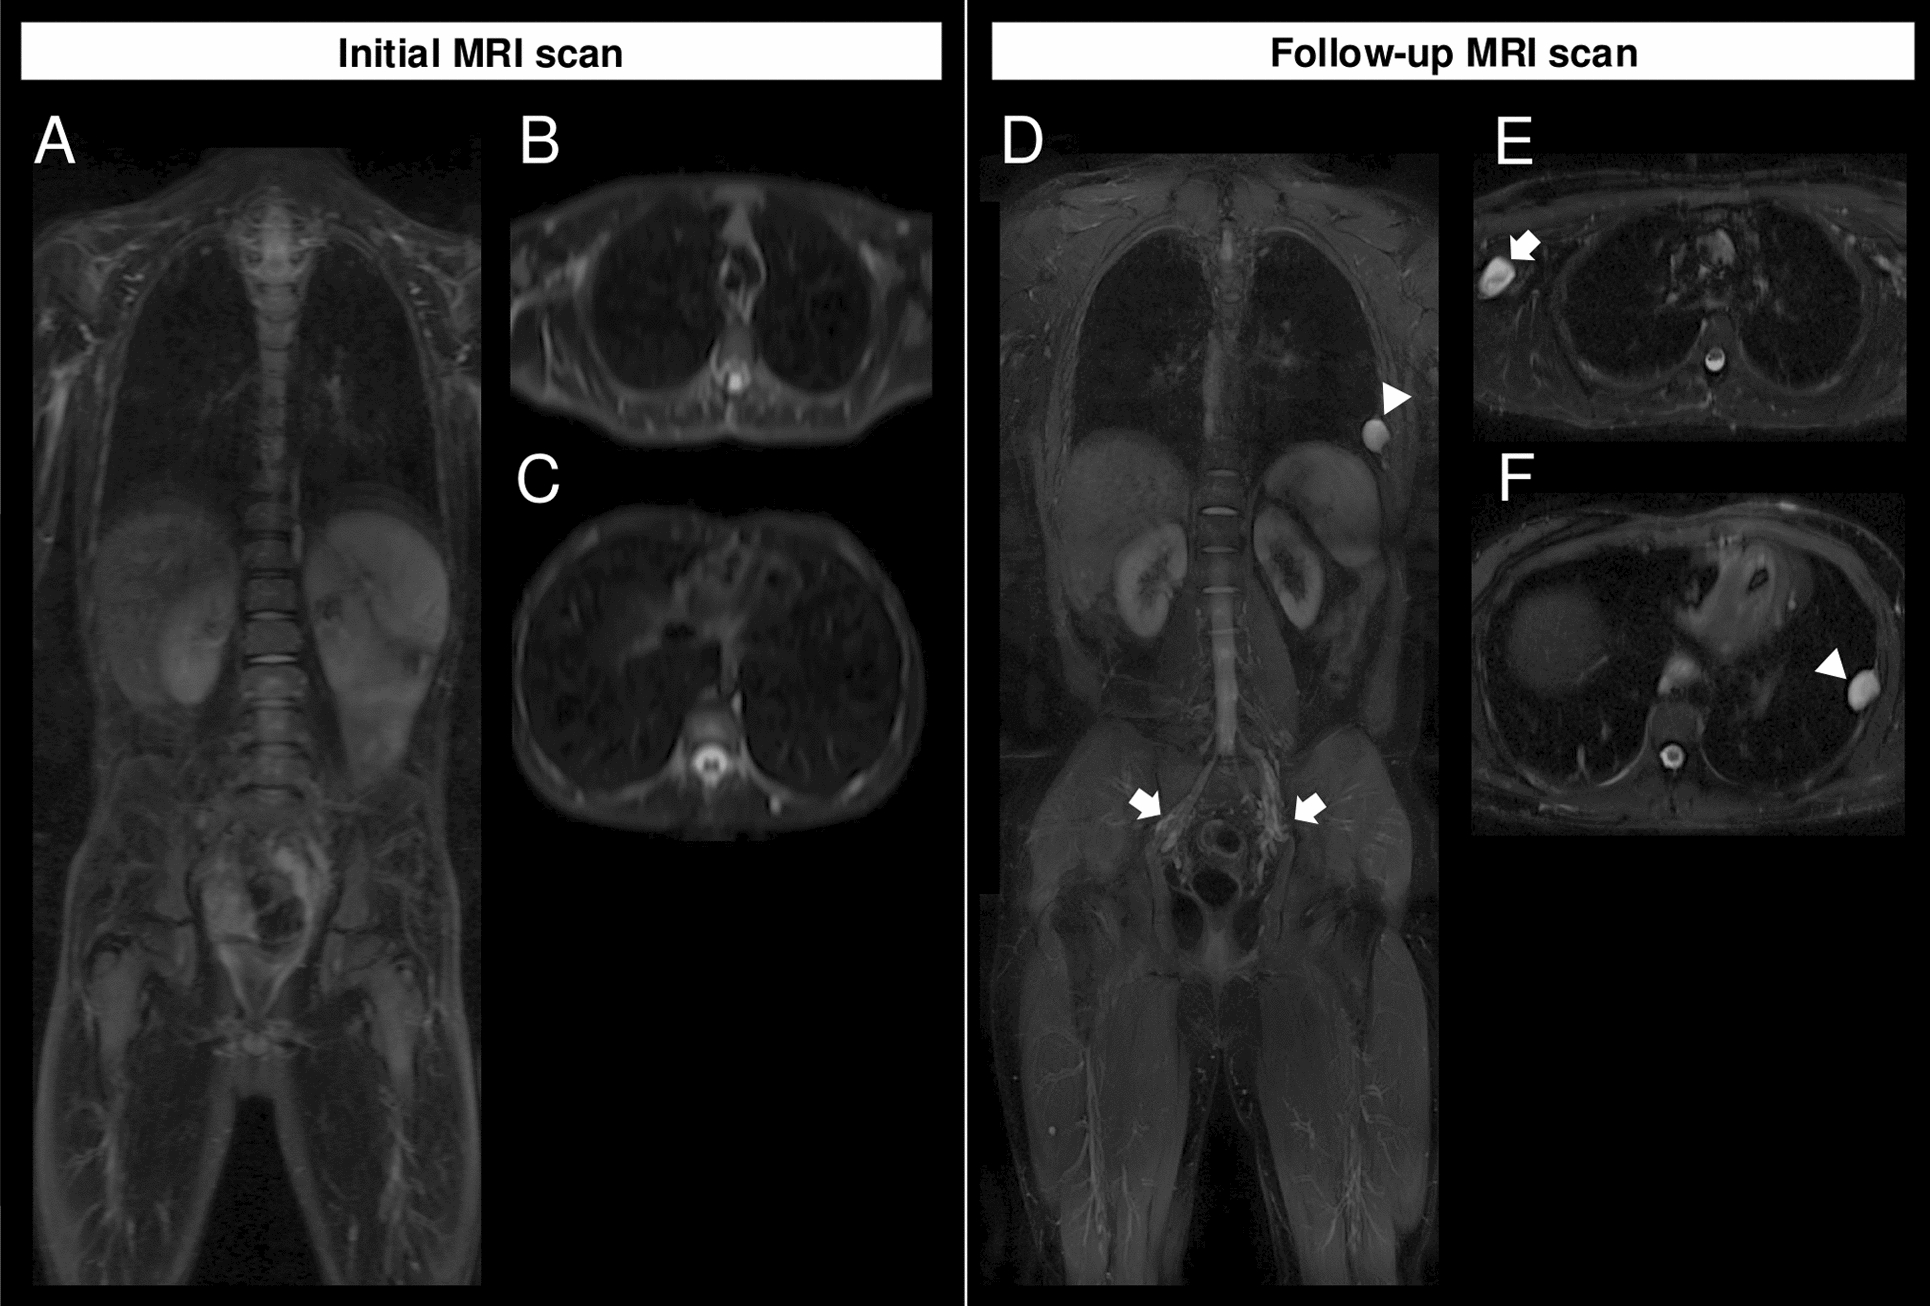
Patient example 1 with newly detected PN during follow-up MRI. A Coronal and B, C axial T2-weighted, fat-saturated MRI sequences of the upper body acquired at 1.5T in a 10-year-old male, who displayed no peripheral nerve sheath tumors at the initial scan in 2007. (D Follow-up examination in 2021, coronal T2-weighted, fat-saturated MRI sequence acquired at 3T at the age of 24, showing a newly developed larger distinct nodular lesion in the left thoracic wall (arrowhead) as well as multiple smaller neurofibromas (arrows). E, F Axial T2-weighted, fat-saturated MRI of the follow-up examination in 2021 with newly developed larger distinct nodular lesions in the left thoracic wall (arrowhead) and in the right upper arm (arrow)
